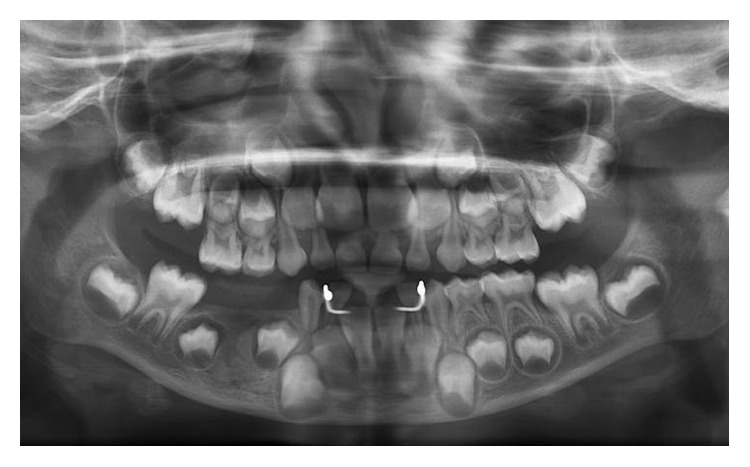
Postoperative panoramic radiograph after 6 months of follow-up with a space maintainer and no evidence of recurrence.
